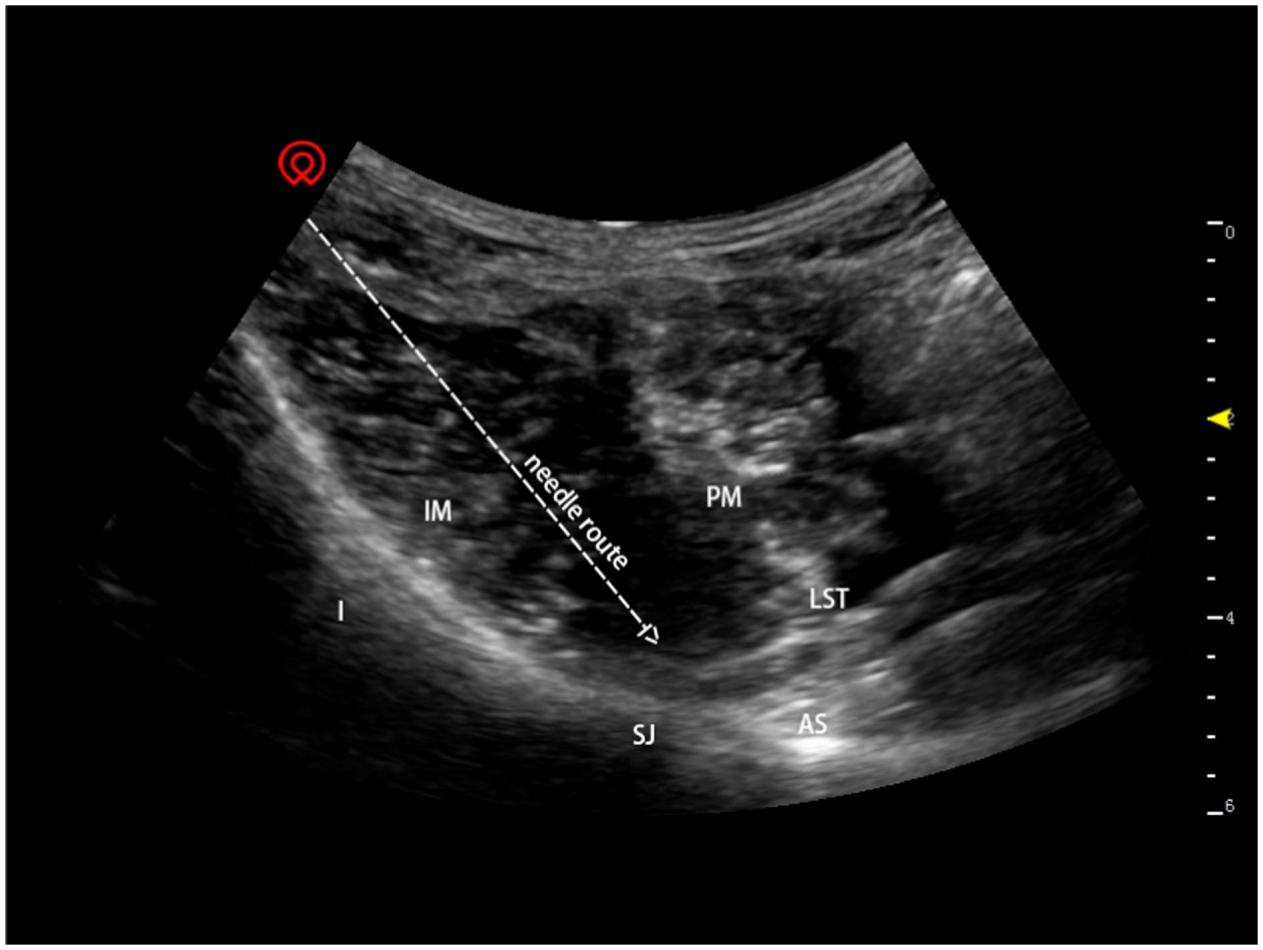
Ultrasound-guided anterior iliopsoas muscle space block. I: ilium; IM: iliac muscle; LST: lumbosacral trunk; PM: psoas muscle; SJ: sacroiliac joint; AS: alae sacralis.
